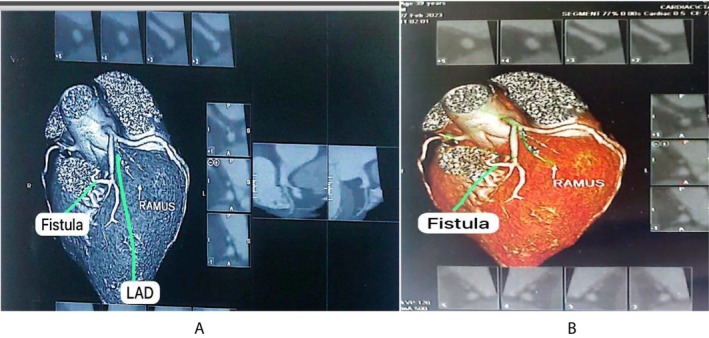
(A and B) Preoperative CT‐angiogram showing an abnormal tortuous branch from mid LAD forming an abnormal fistulous connection with the main pulmonary artery.
